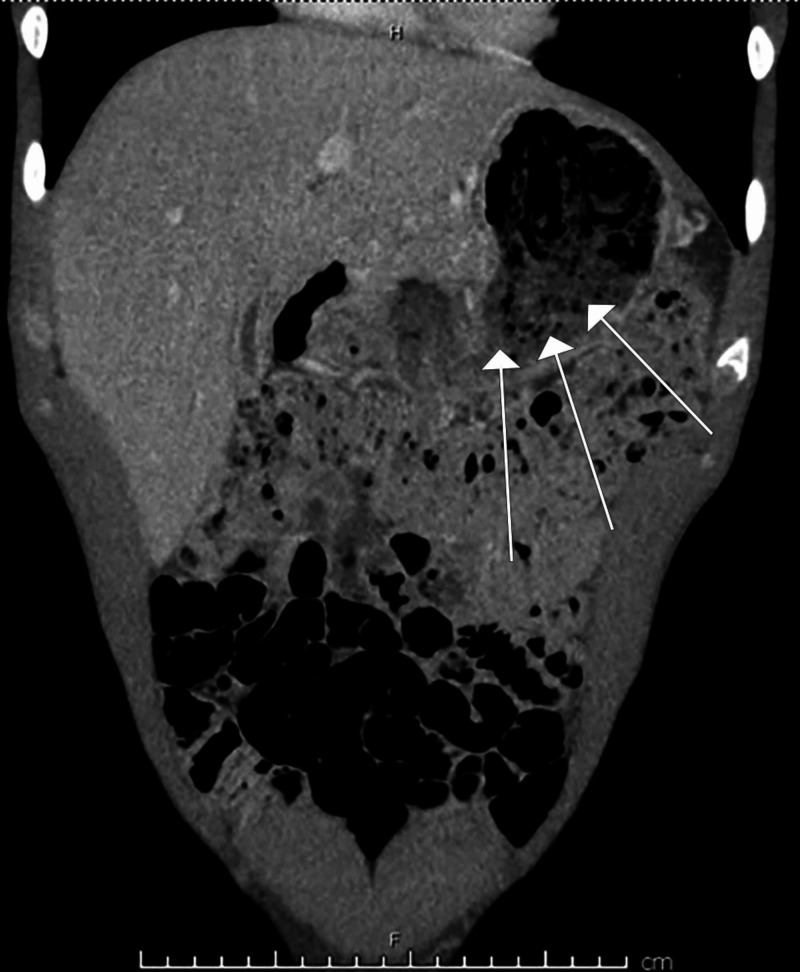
Computed tomography (CT) coronal tomogram demonstrating amorphous material in the stomach compatible with ingested plastic bags (marked with white arrows).
